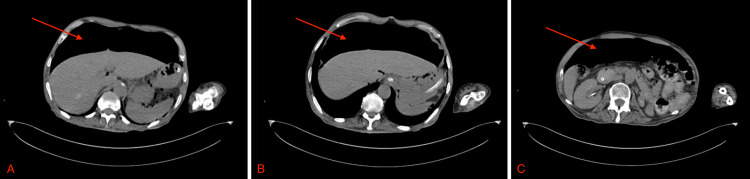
CT-scan showing massive air under the diaphragm at multiple levels, preceding the surgical intervention. CT: computed tomography.
